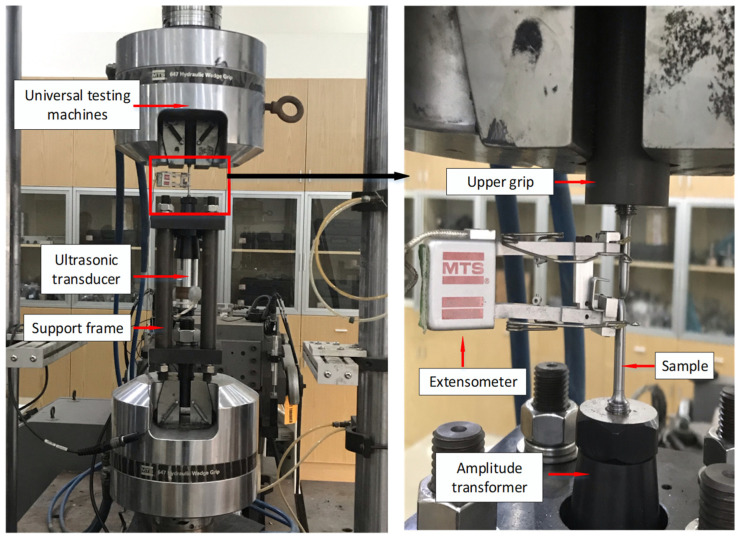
Ultrasonic-assisted tensile test device.
